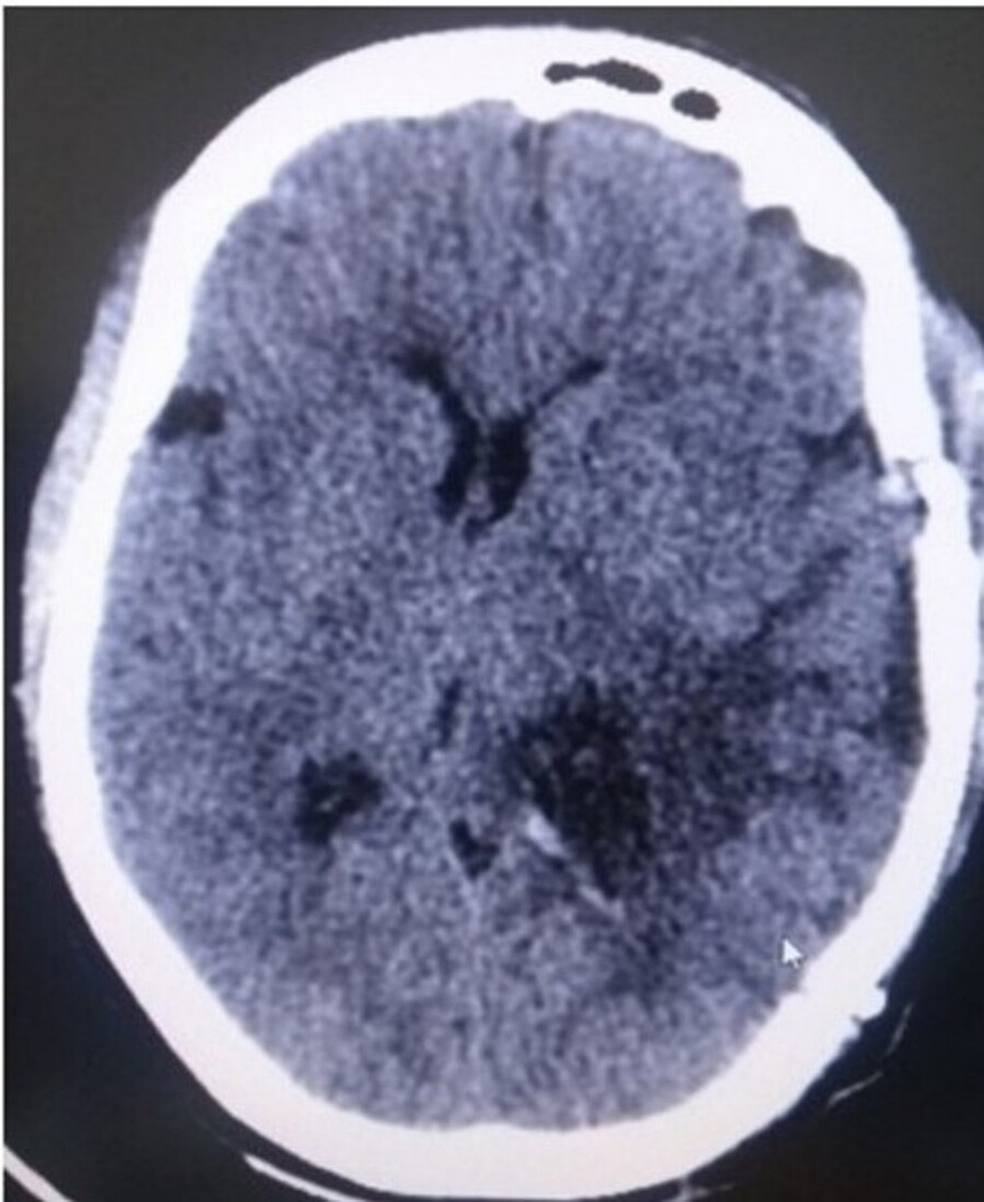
Postoperative CT scan brain - A
